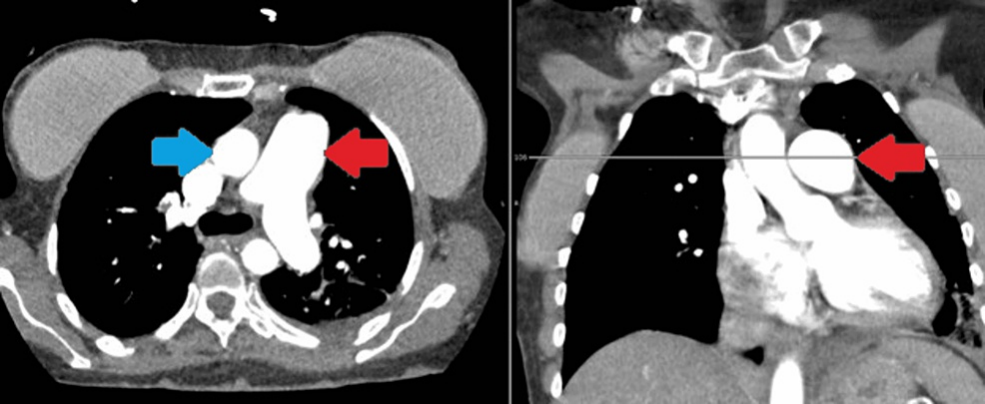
Contrast tomography (CT) of the thorax for case 2 demonstrating enlarged pulmonary arteries (red arrow) in comparison to the ascending aorta (blue arrow).
